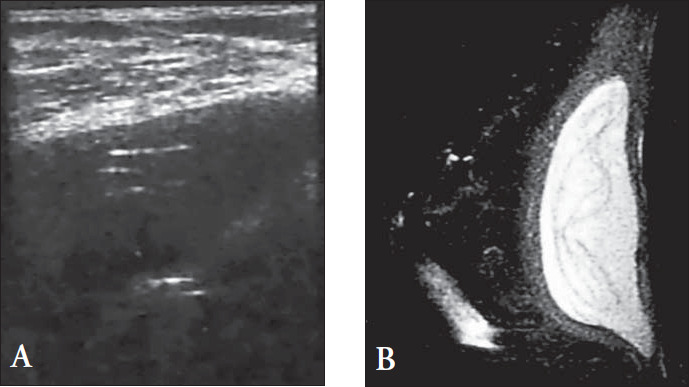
Intracapsular rupture with collapsed elastomer shell. (A) Ultrasonography of contained intracapsular ruptured implant showing multiple layers of the collapsed implant shell visible as sets of parallel echogenic lines (stepladder sign). (B) Sagittal magnetic resonance image showing the same aspect of the collapsed implant shell (linguine sign).
